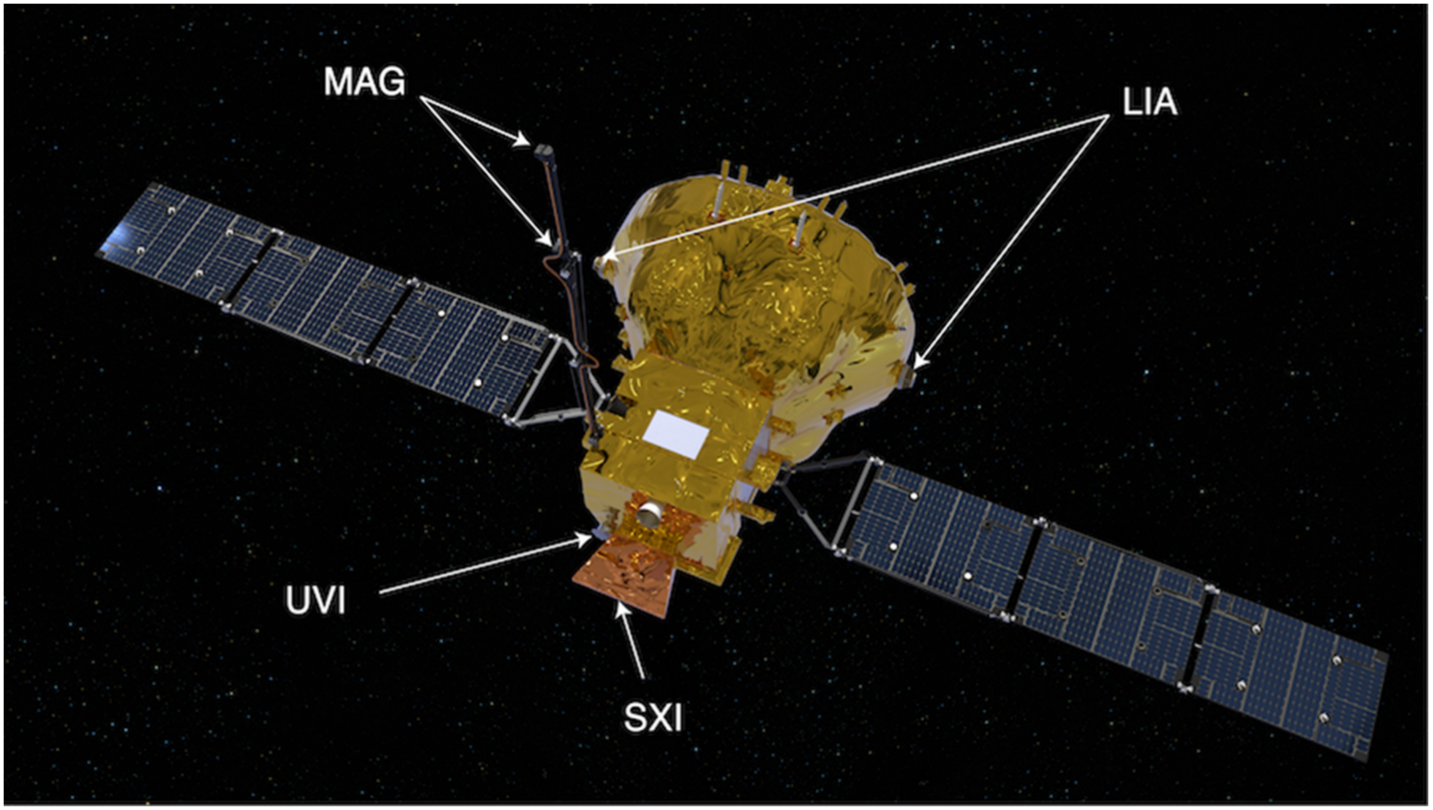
SMILE spacecraft and instruments in flight configuration. The ion instrument two sensors (LIA), magnetometer two sensors (MAG), soft X-ray imager (SXI) and UV imager (UVI) are shown (Work performed by ATG under contract to ESA)
